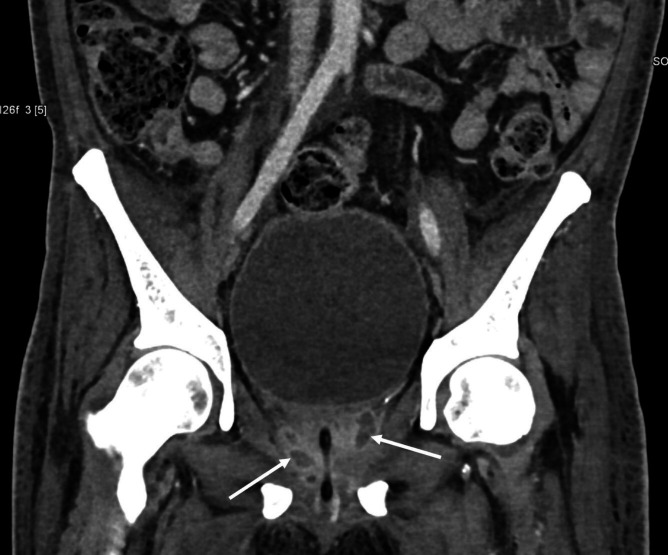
Computed tomography image (coronal view) showing multiple small prostatic abscesses (arrows) in the peripheral zone.
